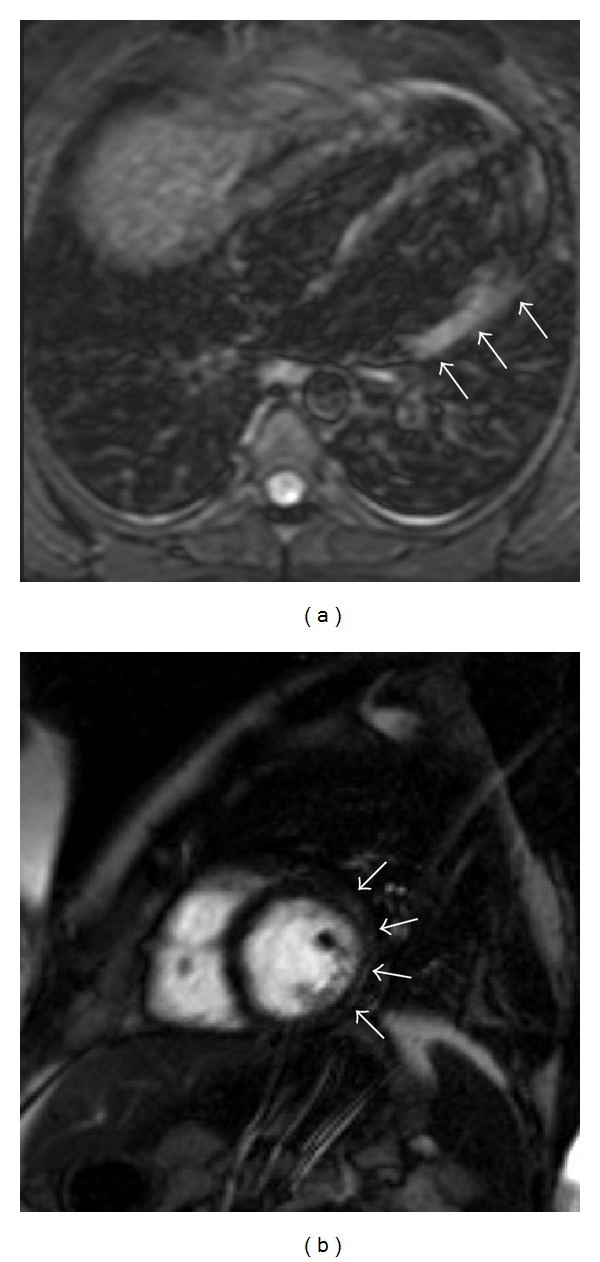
MRI finding of the patient. (a) Short TI inversion recovery (STIR) 4-chamber view showing hyperintense signal of the lateral inferior wall (arrows) suggesting focal edema. (b) Delayed enhancement of midmyocardial short axis showing hyperintense signal on the lateral inferior wall as well as delayed enhancement of the midepicardium and subepicardium (arrows).
